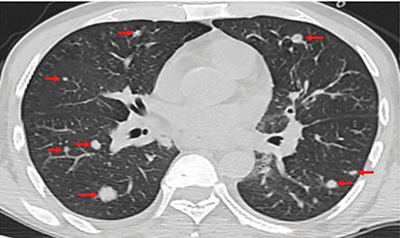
The lung parenchyma shows multiple consolidated nodular areas on non-contrast CT axial slices (red arrows).
